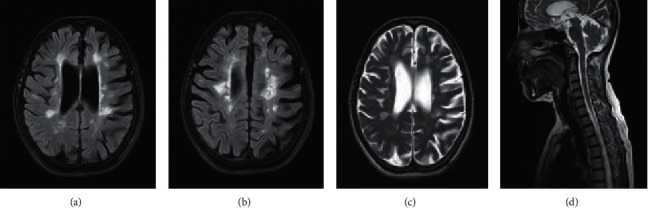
T2 FLAIR axial (a, b) and T2 axial (c) show multiple periventricular Dawson's fingers and juxtacortical lesions. Significant cerebral atrophy is seen with enlarged lateral ventricles suggesting chronic disease. T2 sagittal images of the cervical and upper dorsal spine (d) show multiple short segment lesions without significant cord expansion.
